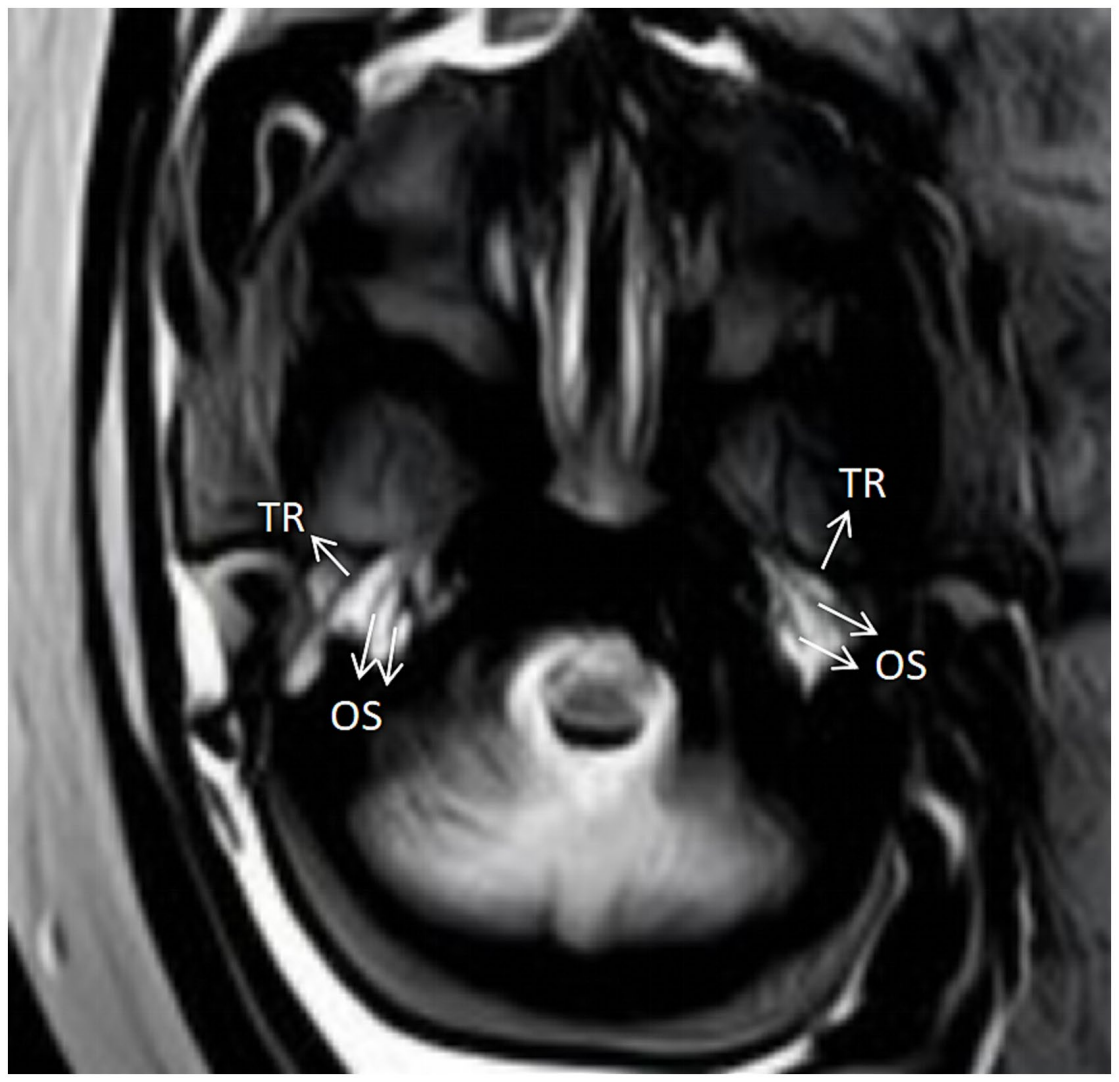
MRI manifestations of the tympanic ring (image acquisition was performed following the method described in Nemzek et al. (10)). TR: Tympanic ring; OS: ossicles.
